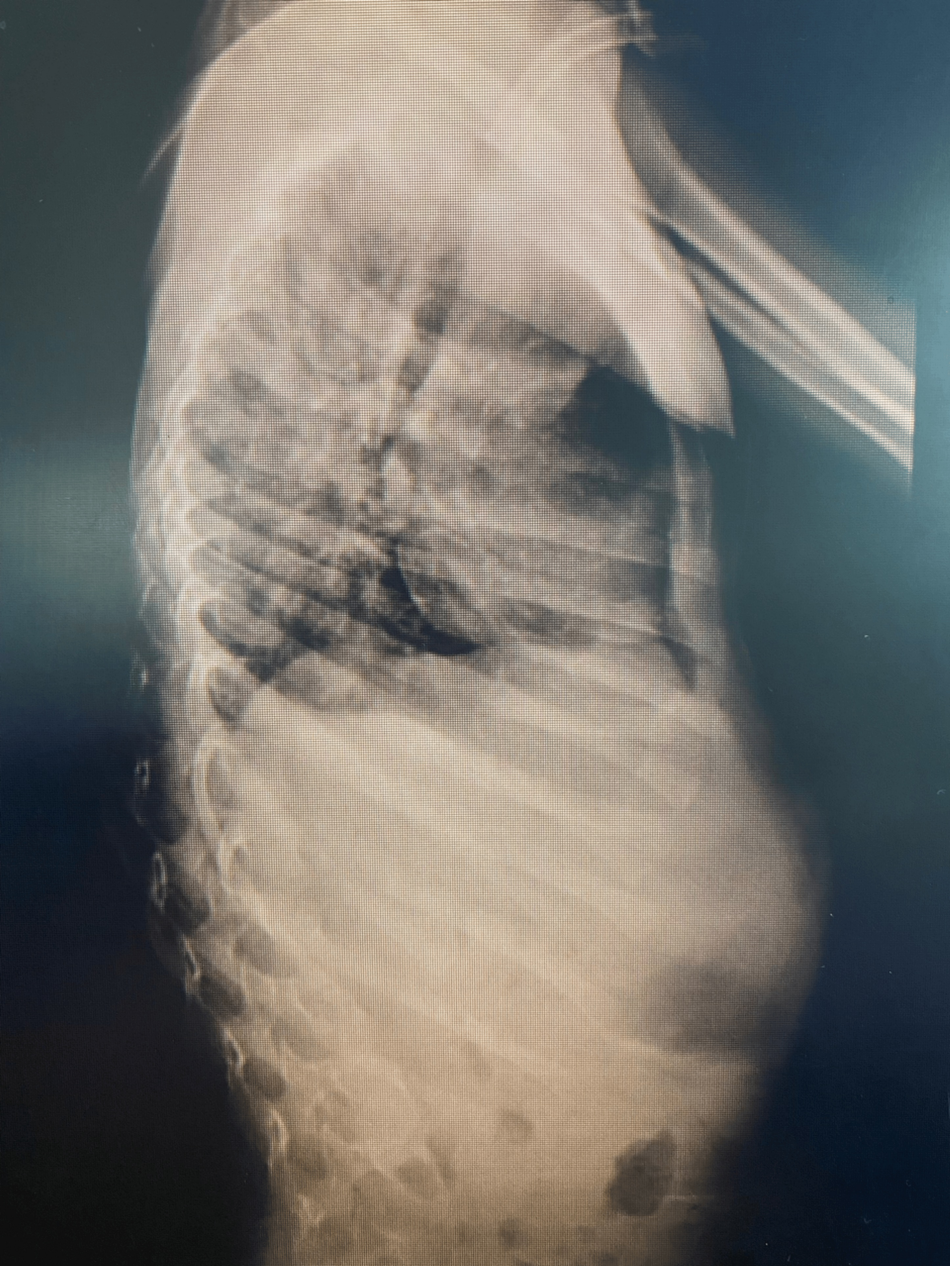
Preoperative lateral view of spine radiograph
